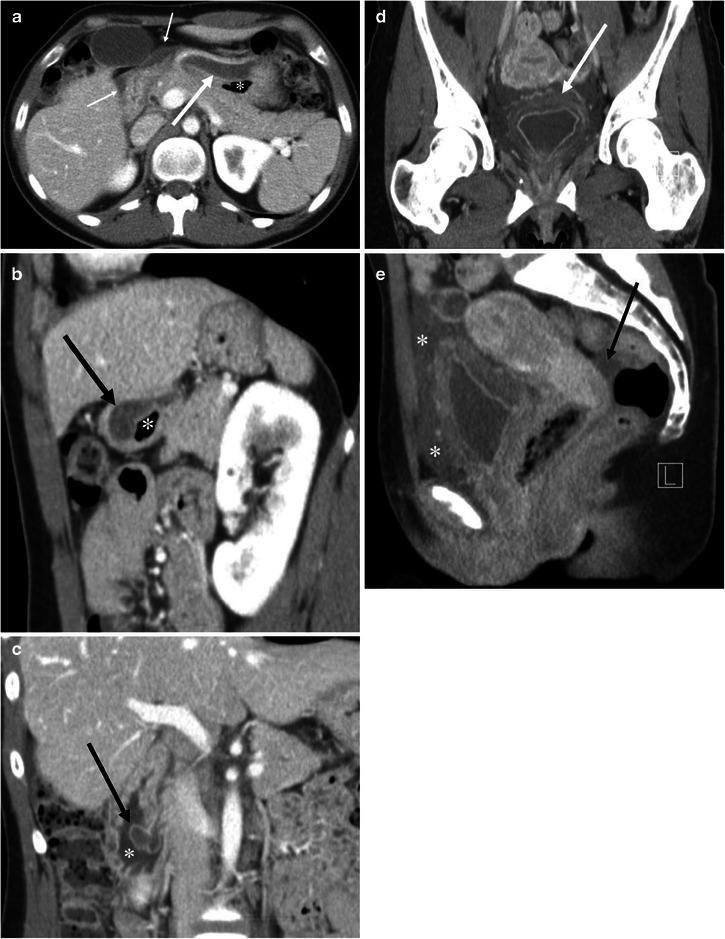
A 27-year-old female with HAE involving the stomach, ampulla of Vater, urinary bladder, and small and large bowel (not shown). a The axial CE-CT shows marked submucosal oedema of the anterior wall of the stomach (*arrow*). *Asterisk* indicates the lumen of the stomach. Fluid is noted adjacent to the stomach and duodenum (*small arrows*). b The sagittal reformatted image clearly shows asymmetrical gastric wall involvement of angioedema (*arrow*). *Asterisk* indicates the lumen of the stomach. c Coronal reformatted image shows marked oedematous swelling of the ampulla of Vater (*arrow*). *Asterisk* indicates the duodenal lumen. d The coronal reformatted image of the pelvis shows mucosal enhancement and extensive submucosal oedema of the urinary bladder (*arrow*). Urinalysis was unremarkable without evidence of urinary tract infection. e The sagittal reformatted image shows fluid around the urinary bladder in the extraperitoneal space (*asterisks*). *Arrow* indicates a small amount of intraperitoneal free fluid in the cul-de-sac
